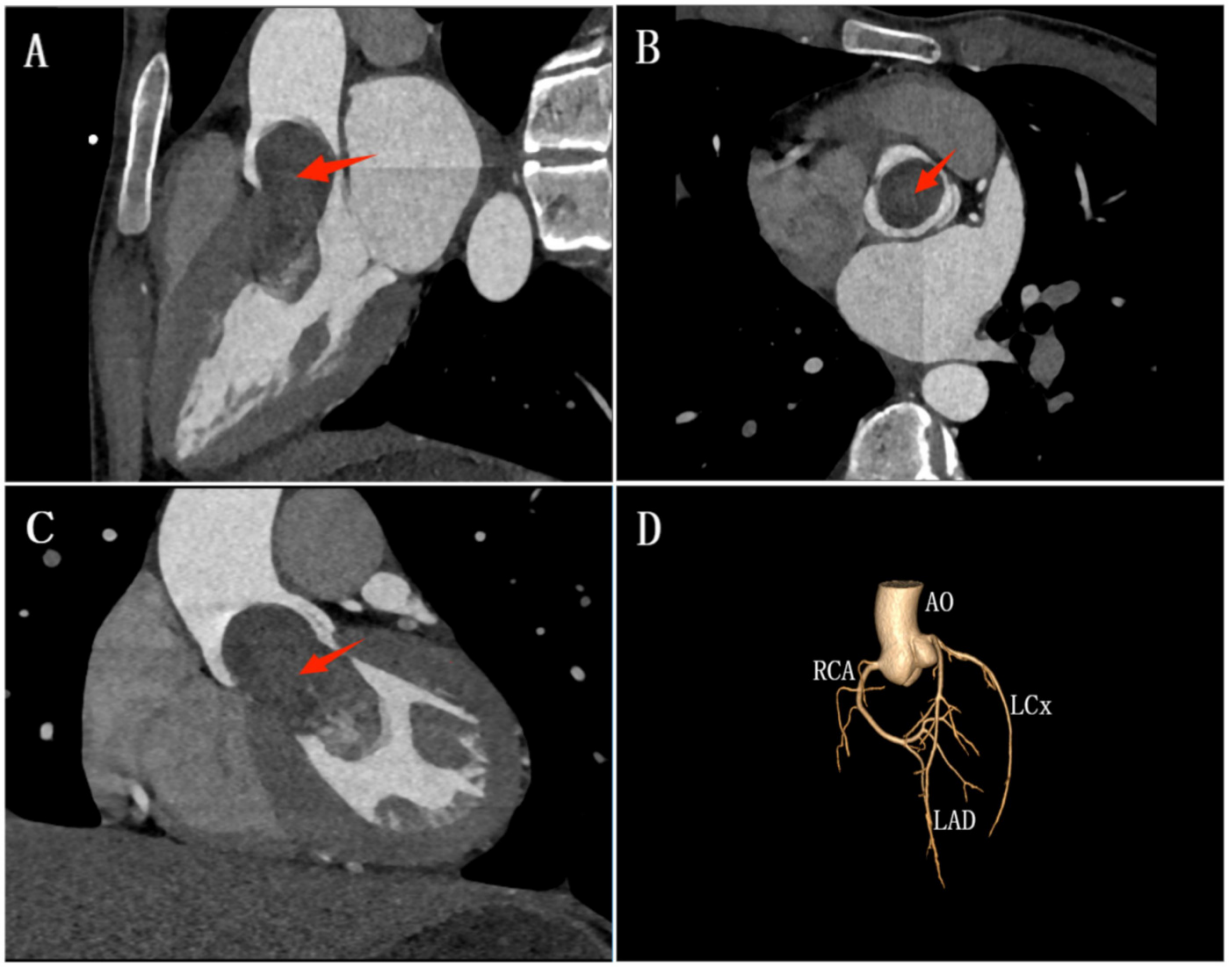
CTA imaging of the left ventricular fibroma causing dynamic LVOT obstruction. Preoperative CTA offered detailed visualization of the left ventricular fibroma across sagittal, horizontal, and coronal planes (red arrow), revealing its dimensions measuring approximately 5.22 cm × 2.48 cm × 2.34 cm (A–C). Subsequent 3D coronary reconstruction clearly delineated all branches, revealing no evidence of vascular compression or invasion by the fibroma and no signs of blood flow obstruction (D).
